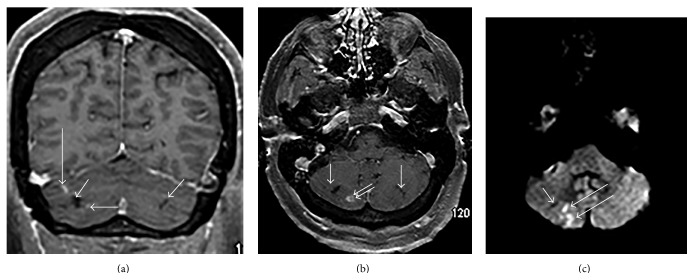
(a) Coronal postcontrast T1 weighted magnetic resonance (MR) image shows areas of old (chronic) infarction in the equators of both cerebellar hemispheres (short white arrows) and small, enhancing subacute infarcts in right cerebellum (long white arrows). (b) Axial postcontrast T1 weighted MR image shows areas of old (chronic) infarction in both cerebellar hemispheres (single white arrows) and an enhancing subacute infarct in the right cerebellum (double white arrow). (c) Axial diffusion weighted MR image (DWI) through the posterior fossa shows a small, old right cerebellar infarct (short white arrow) and areas of subacute infarction with diffusion restriction (long white arrows).
